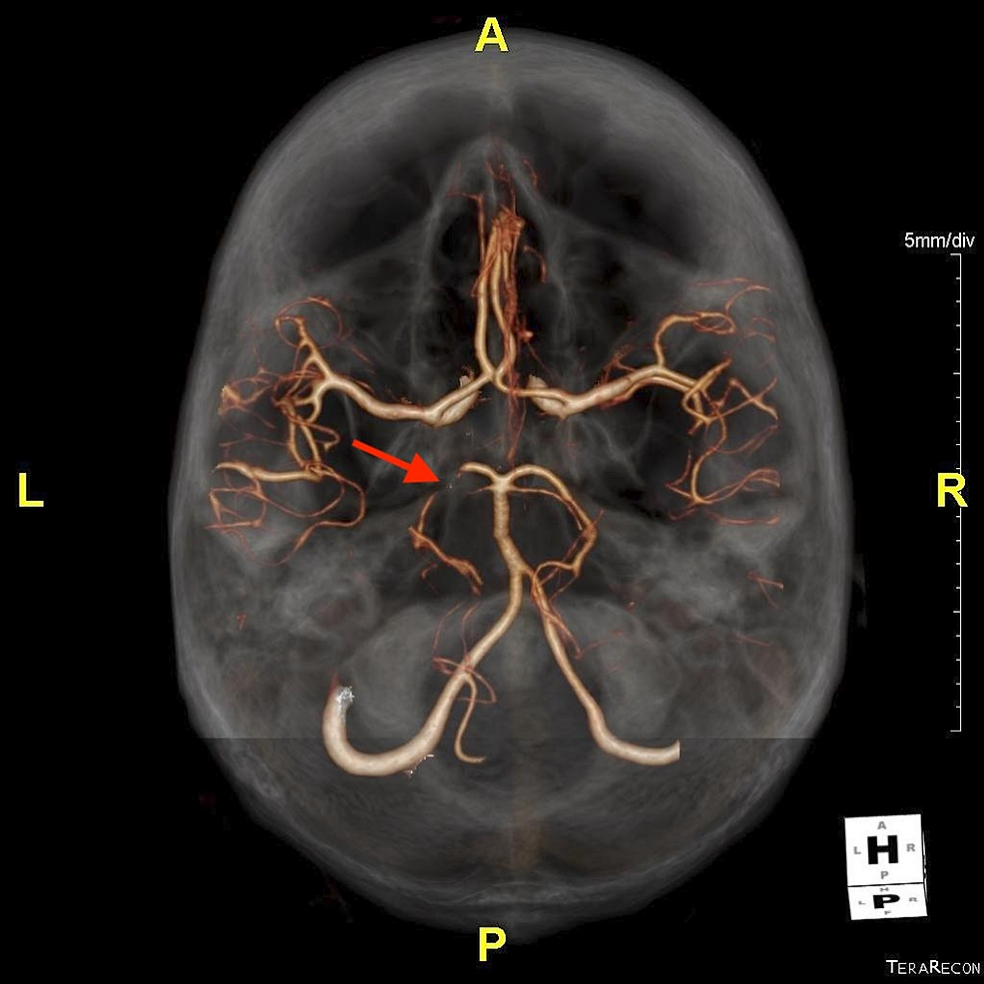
CT angiogram of the head demonstrating severe stenosis of the proximal left PCA. CT: computed tomography; PCA: posterior cerebral artery
